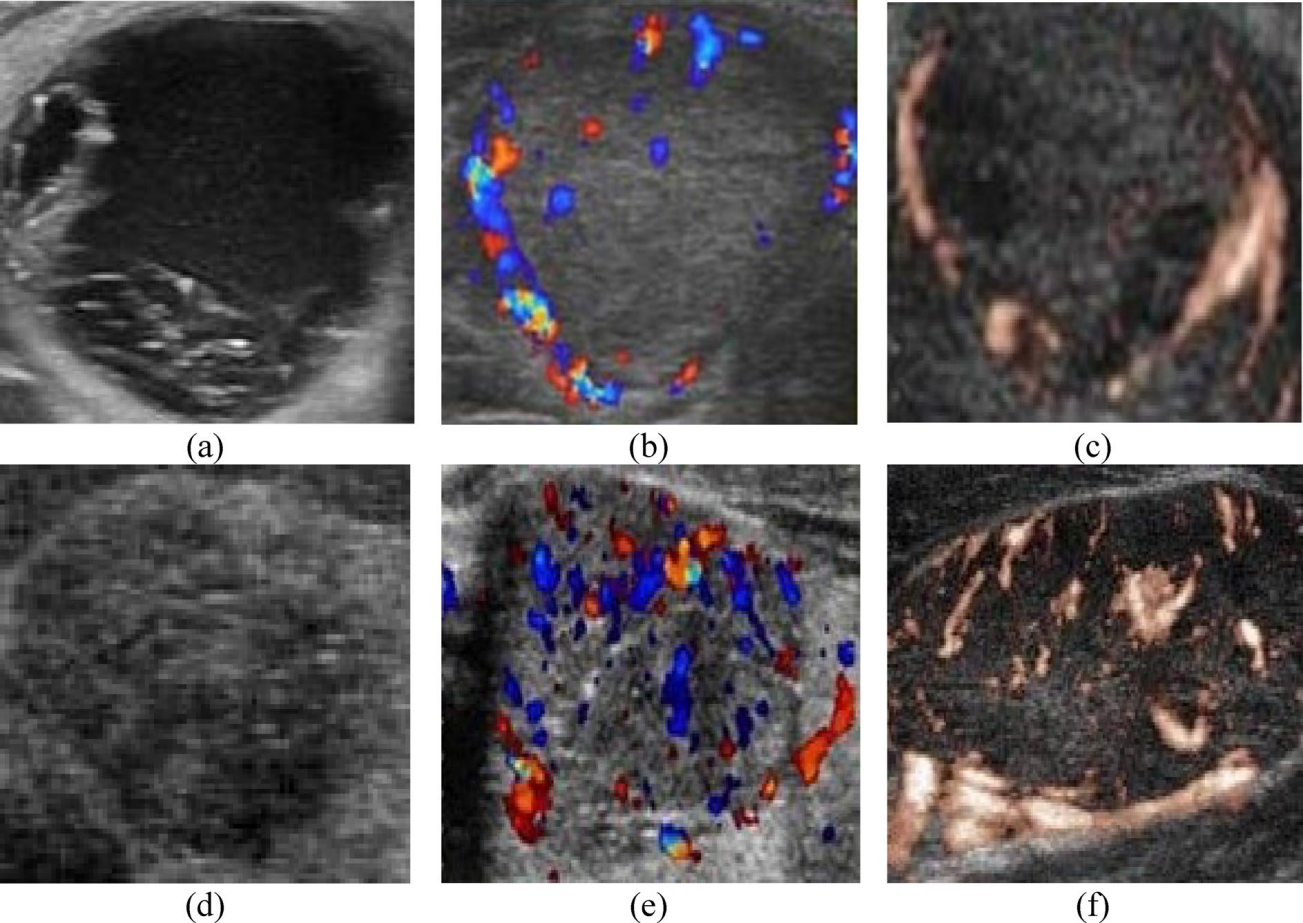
A representative sample from the ultrasound cases dataset. (a) Gray scale benign TN image. (b) Color doppler benign TN image. (c) Power doppler benign TN image. Likewise (d) & (e) & (f) are three malignant TN images.
